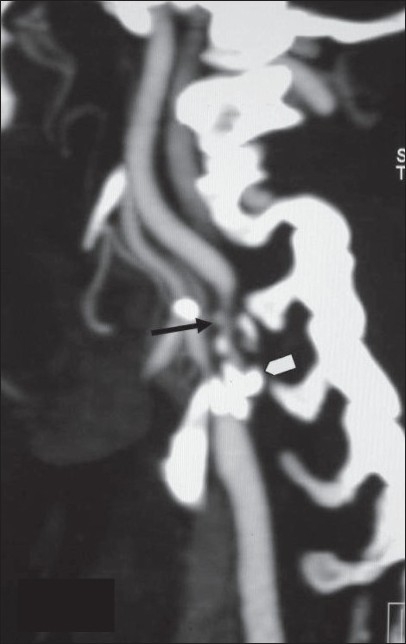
Saggital MIP image of a CT angiogram shows significant ICA narrowing (arrow) due to a calcified plaque (arrowhead) in the bulb and at the origin
